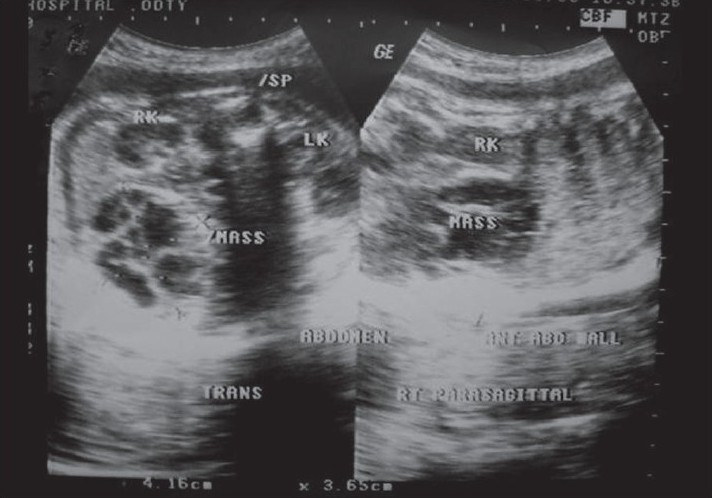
Ultrasound showing complex ovarian cyst
